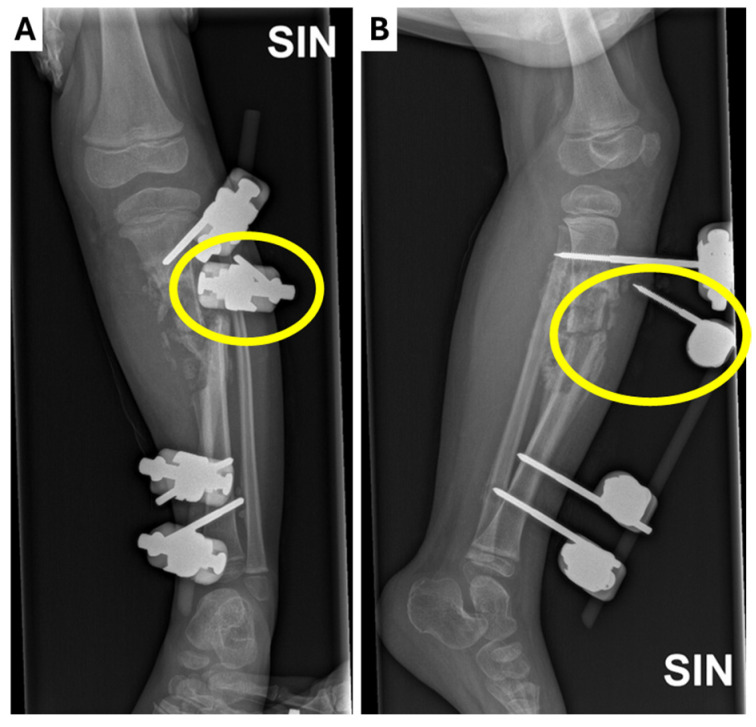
Radiological examinations of the left leg showing the dislocation of one of the external fixators (yellow circle) in the (A) X-ray anterior–posterior projection; (B) X-ray lateral projection.
